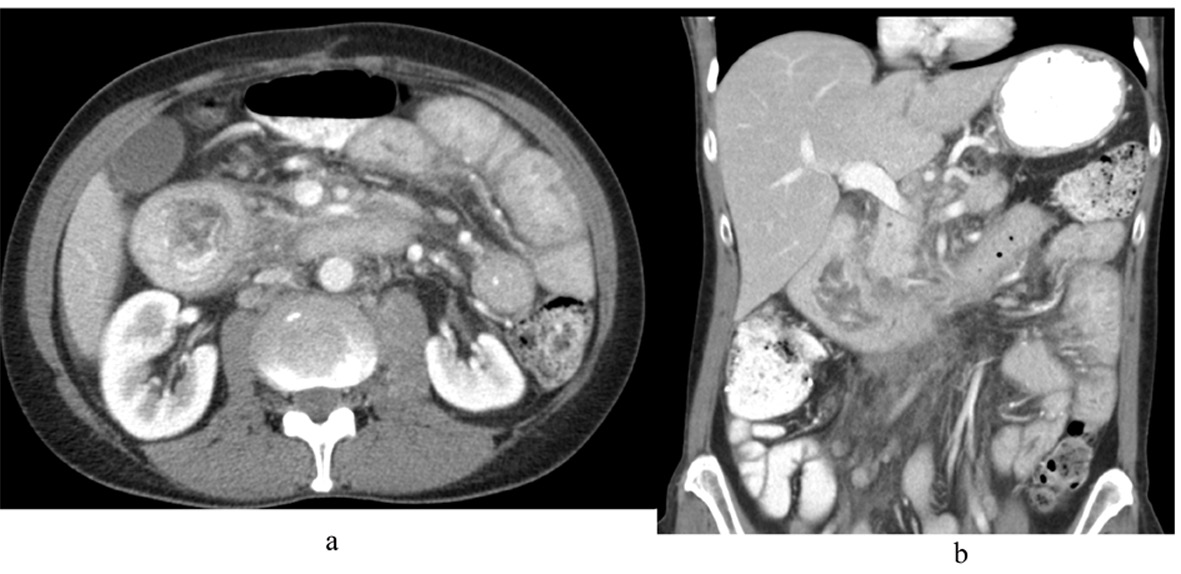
CT scan of abdomen and pelvis with IV contrast (A) axial view and (B) coronal view showing marked distention with irregular wall thickening of the duodenum just proximal to the genu causing a partial gastric outlet obstruction.
